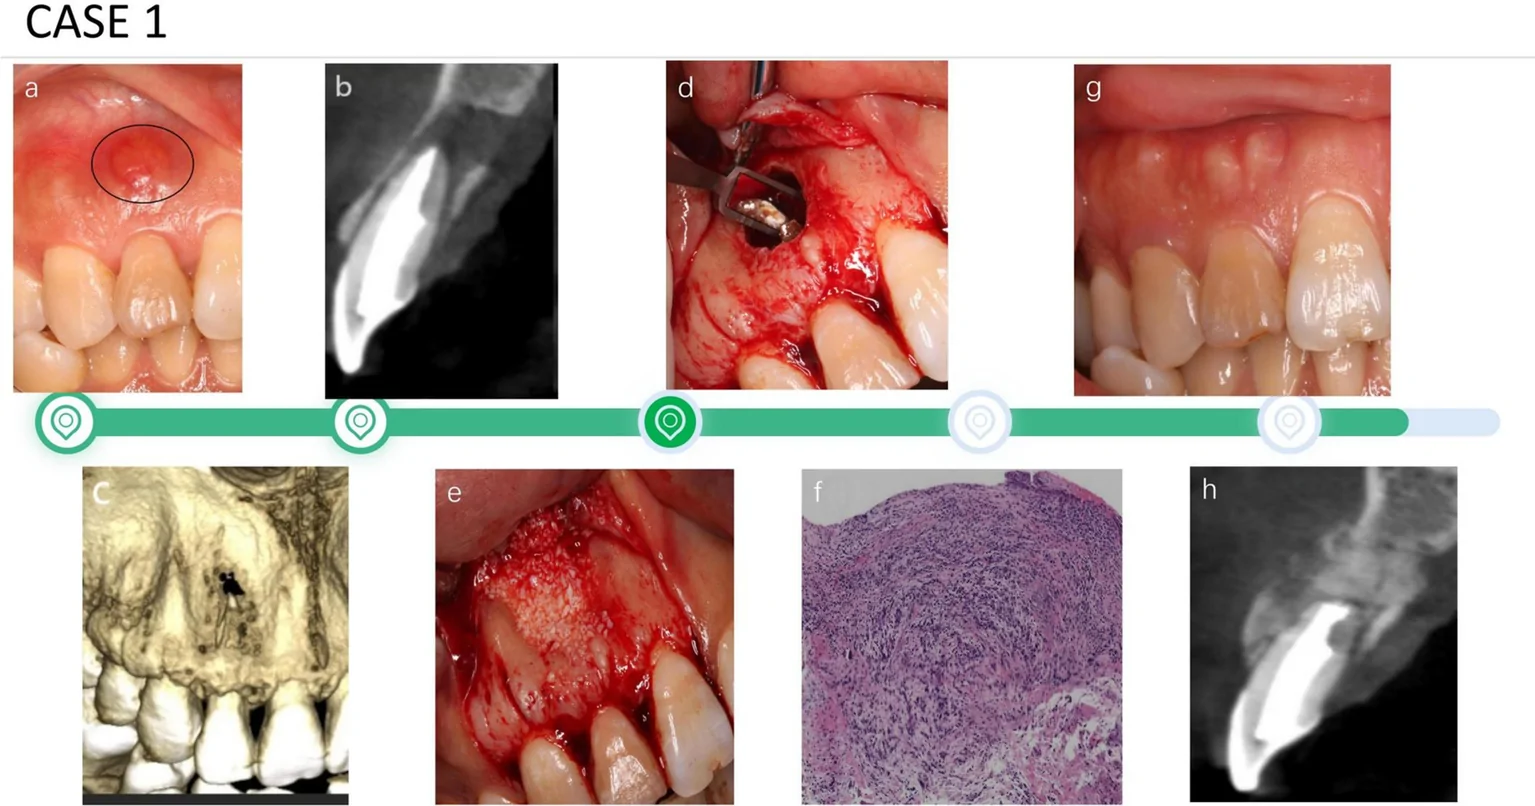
Case 1: Tooth#7 involving penetration of the labial cortical bone. (a) The initial intraoral photograph showing the buccal mucosa near tooth#7 is red in the circular. (b,c) A large apical radiolucency encompassing tooth#7 and the large defect of cortical bone in CBCT. (d) After flap elevation and lesion curetting, root tip resection, and sealing of the root tip with iRootBP Plus. (e) The apical bone defect of tooth#7 was restored with GBR. (f) Inflammatory cells consisting of lymphocytes, plasma cells, and histiocytes in the epithelium of the radicular cyst. (g) A sinus tract disappearing and the gingiva in good condition at the 12-month follow-up. (h) 12-month follow-up CBCT axial view slices showing complete healing.
